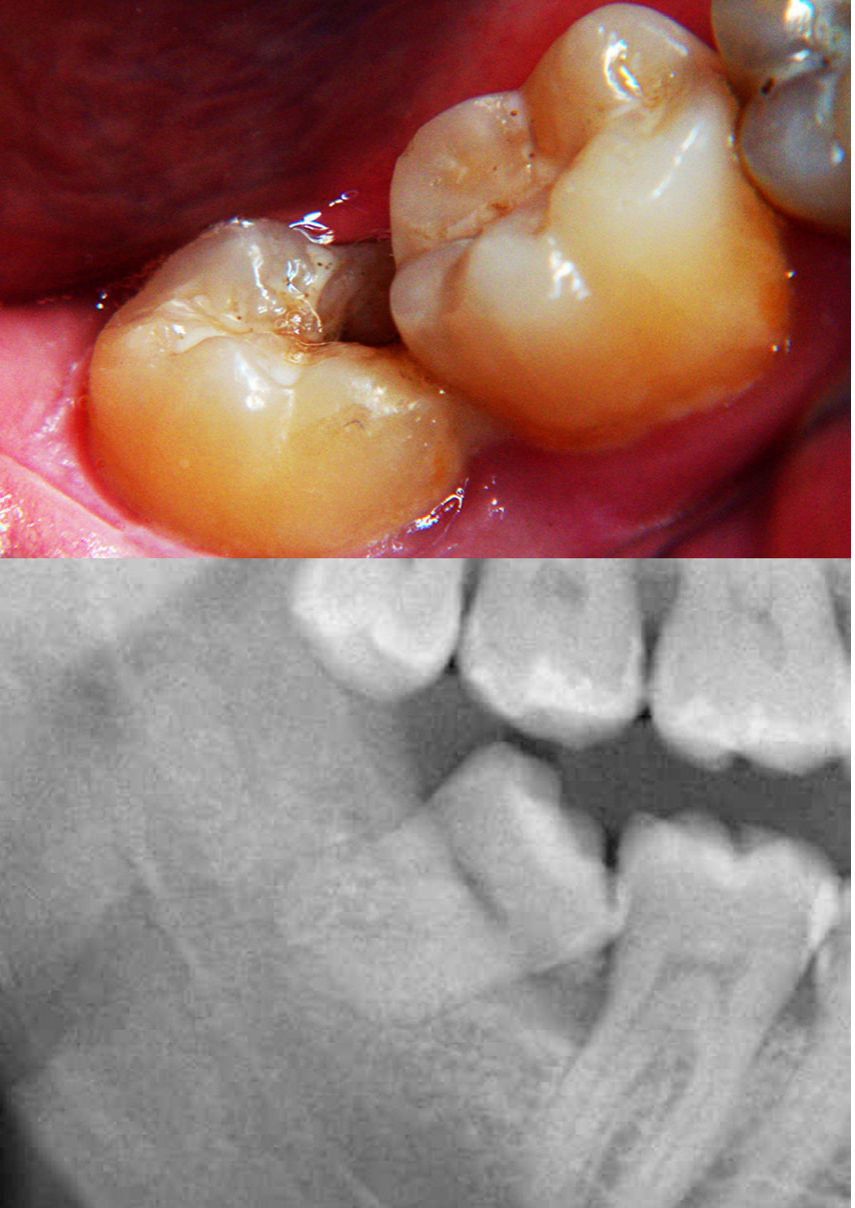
(a) Intraoral view showing defect in the mesial wall of the third molar associated with bony defect distal to the second molar and local erythema. (b) Preoperative radiographic image showing mesially impacted third molar in contact with the distal surface of the second molar causing radiolucency at the contact point (dental decay). There is also slight periradicular radiolucency (inflammation) and dilation or thickening of the lamina dura.
